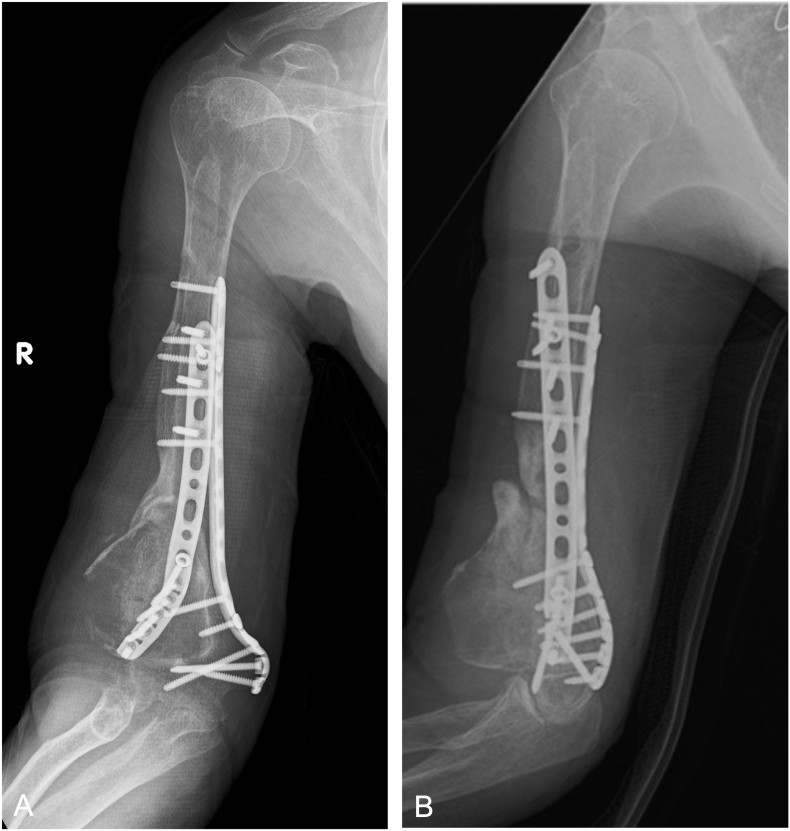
Postoperative X-ray findings. A) Anterior posterior view of the humerus. B) Lateral view of the humerus.
